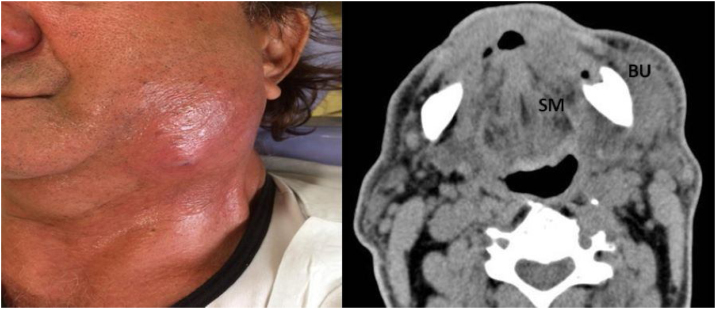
Case # 1. A 74-year-old man without comorbidities presented with an odontogenic infection of the left posterior lower teeth and who was hospitalized for 3 days. (a) Left front view revealing swelling in the lower left third of the face; (b) Contrast enhanced CT revealing a collection in the homolateral Submandibular (SM) and Buccal (BU) spaces.
